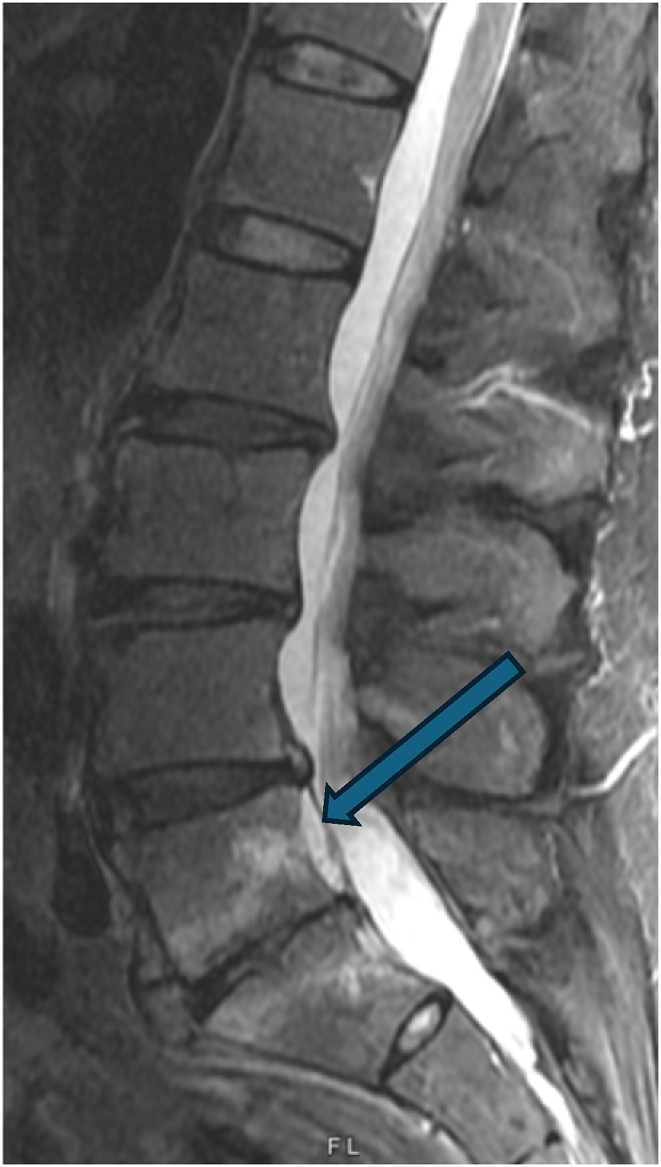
Sagittal STIR MRI 11 months post-procedure. Arrow demonstrates a smaller hematoma posterior to the L5 vertebral body.
